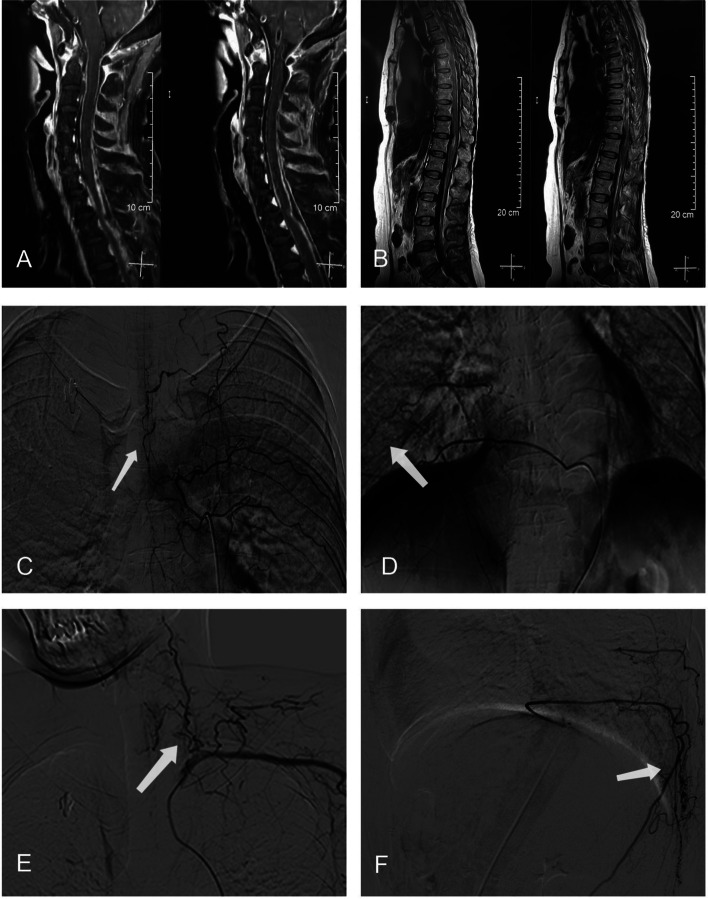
Spinal cord MRI showed subarachnoid haemorrhage in the cervicothorax and waist segments (A and B). Digital subtraction angiography showed beaded changes of spinal cord vessels in the thoracic spinal cord and lower cervical spinal cord (C, D, E and F) (arrows)
